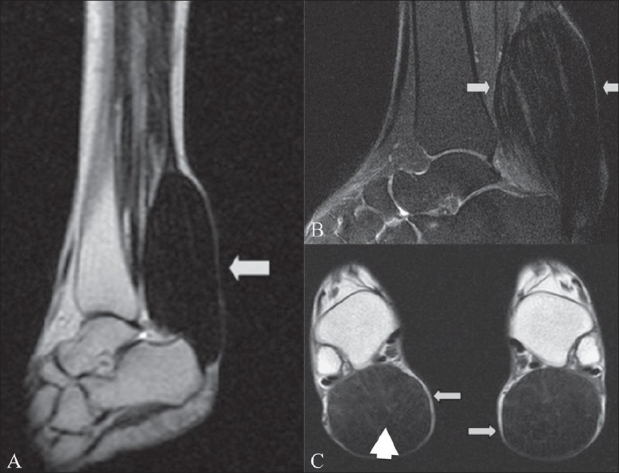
T2W sagittal (A), proton density fat-suppressed sagittal (B) and T1W axial (C) MRI images of the ankles and Achilles tendons show enlarged bilateral Achilles tendons showing classic hypointensities (arrows). A few linear isointense to hyperintense linear lesions are seen within (arrowheads), giving rise to a slightly reticular appearance
